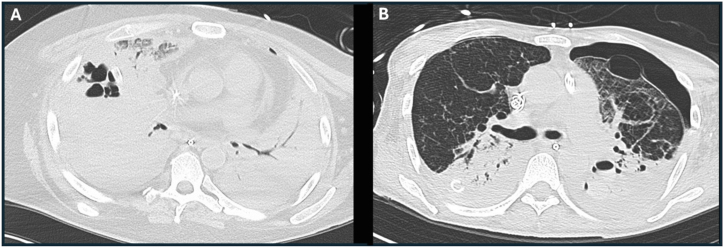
Representative computed tomography (CT) images of two clinical cases of necrotizing pneumonia. A. A non-contrast CT chest image from the patient described in case 5. Extensive consolidation of the bilateral lower lobes and multiple cavities in the right lower lobe with air-fluid levels are seen. B. A non-contrast CT chest image from the patient described in case 6. The scan is remarkable for bilateral lower lobe consolidation, a chest tube in the right lower lobe, and a hydropneumothorax and cavitary lesions with air-fluid levels in the left lower lobe. The hydropneumothorax was sampled by thoracentesis, and the pleural fluid culture grew *P. aeruginosa*.
